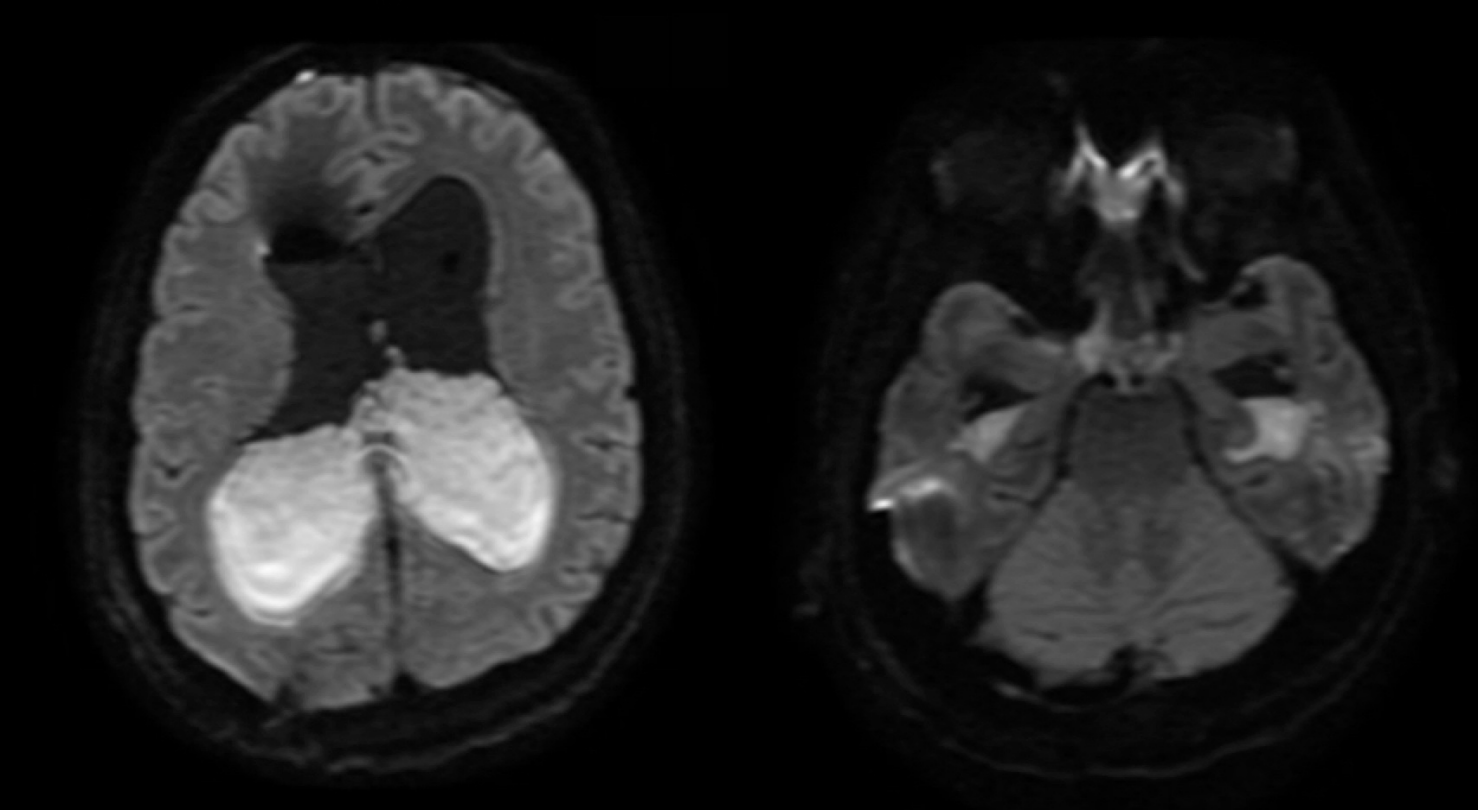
Magnetic resonance imaging (MRI) obtained (axial diffusion weighted imaging) at Day 4 of infection was notable for extensive infection with ventriculitis and basilar meningitis with diffuse subarachnoid pus throughout the basilar cisterns
